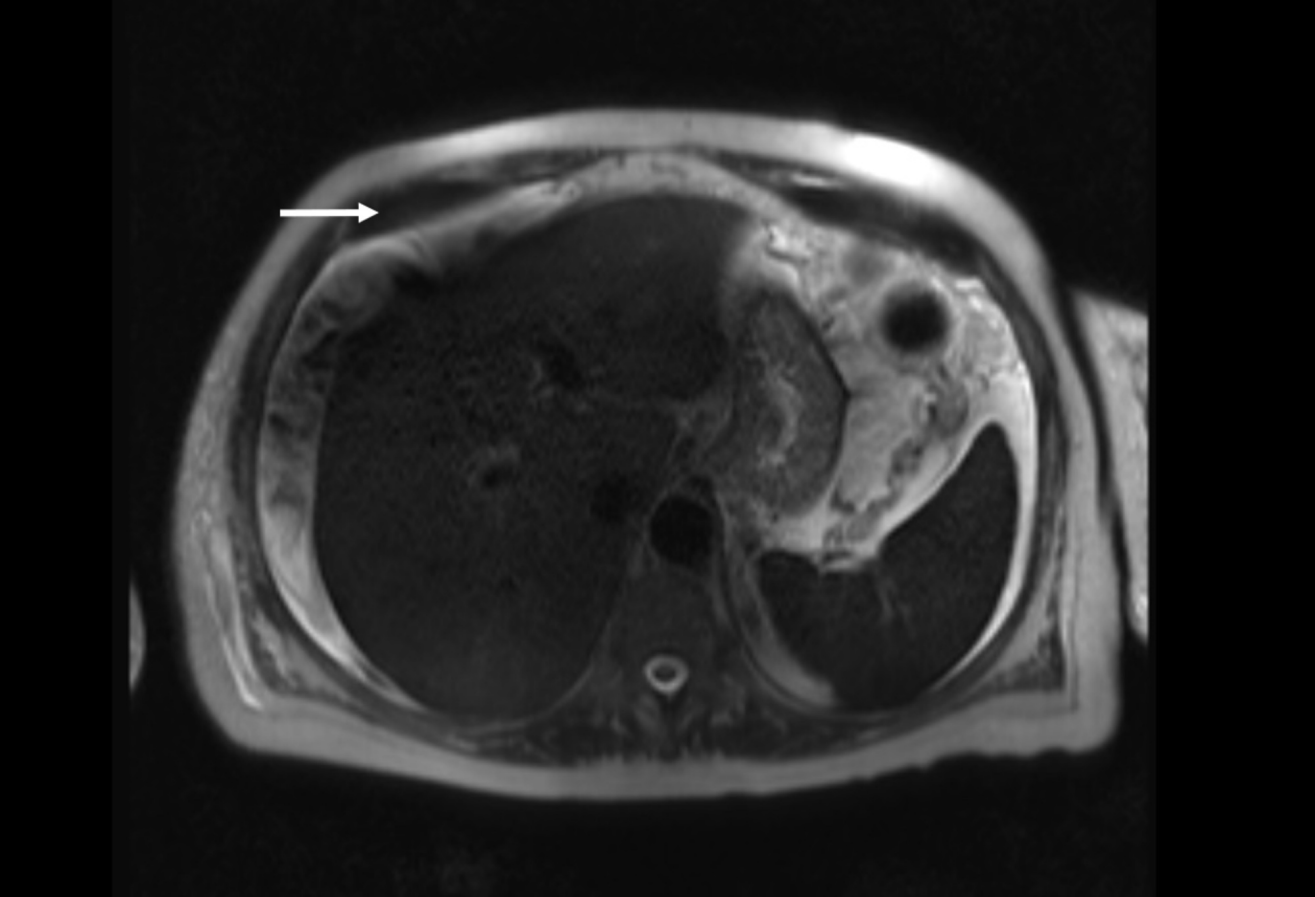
Abdominal MRI Normal appearing liver with a moderate volume of ascites
